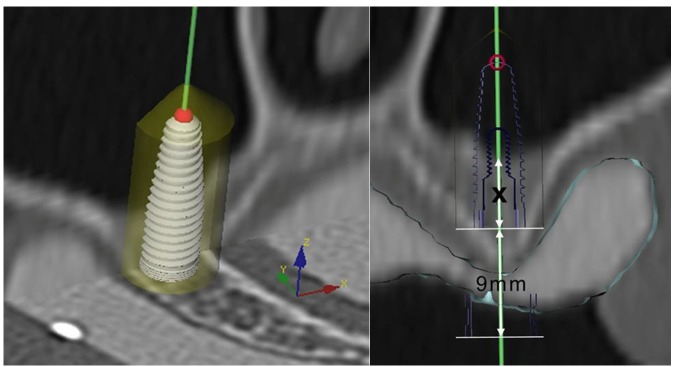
3D planning procedure for flapless transcrestal sinus floor elevation on preoperative CT scans to determine the correct drilling depth to avoid perforation of the maxillary sinus membrane.
